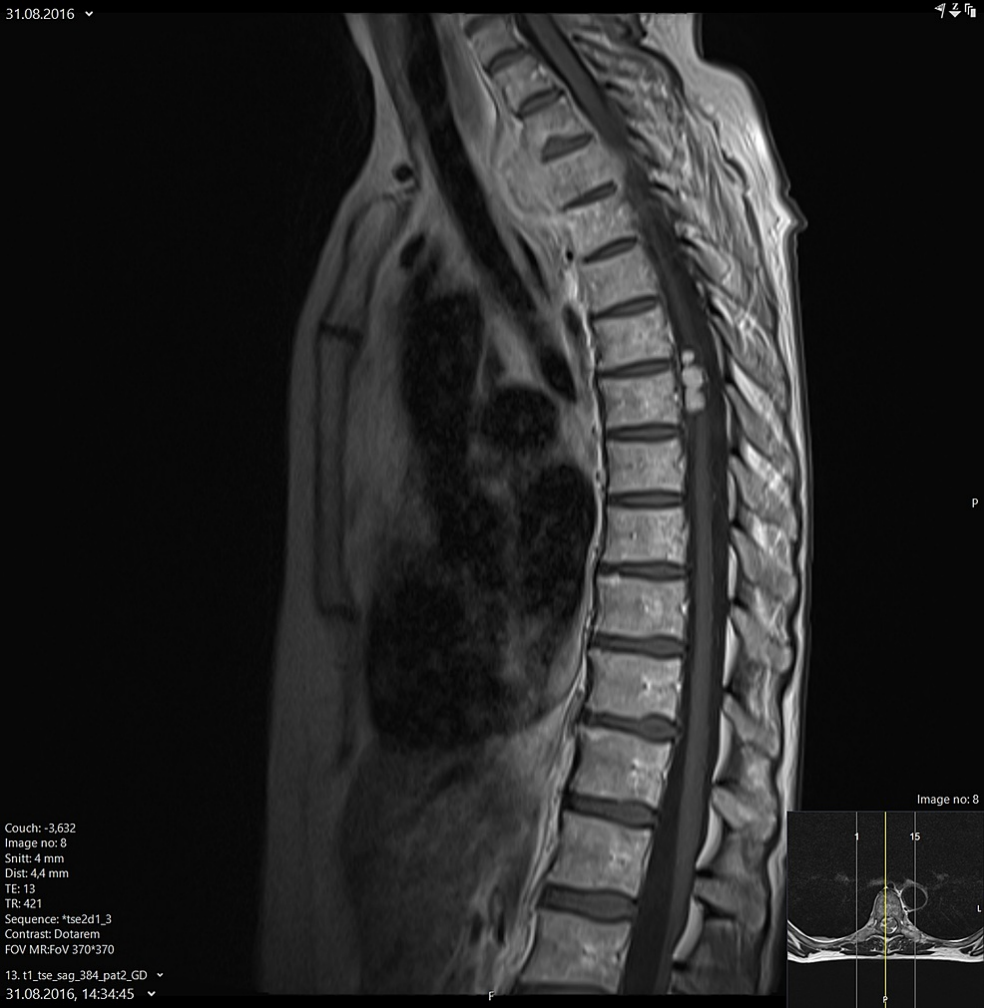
Sagittal contrast-enhanced T1 TSE MRI scan of the intraspinal lesion. MRI, magnetic resonance imaging; TSE, turbo spin echo
